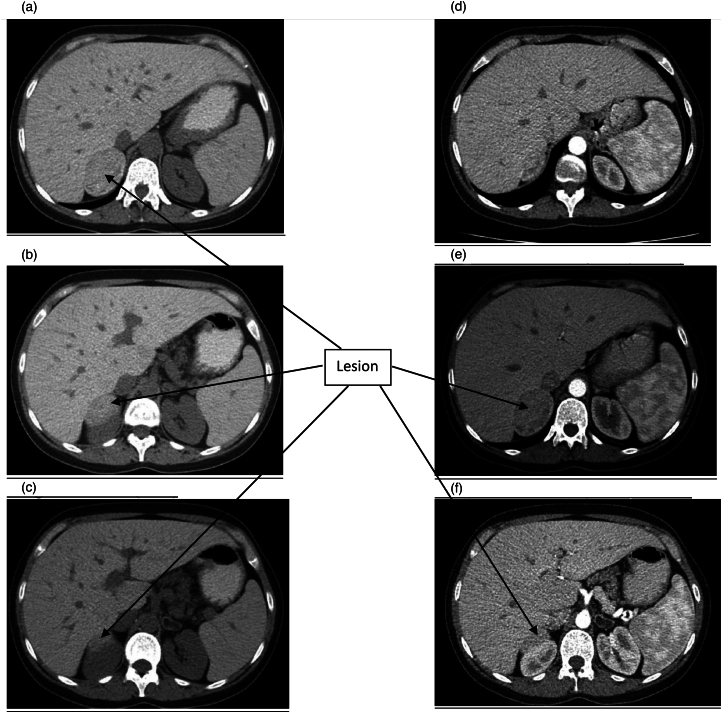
Computed tomography scans: (a) no contrast (NC) (H=−65), (b) NC (H=−80), (c) NC (H=−85), (d) arterial phase H=−58.5, (e) arterial phase H=−73.5, (f) H=−92.9. H = Hounsfield units.
